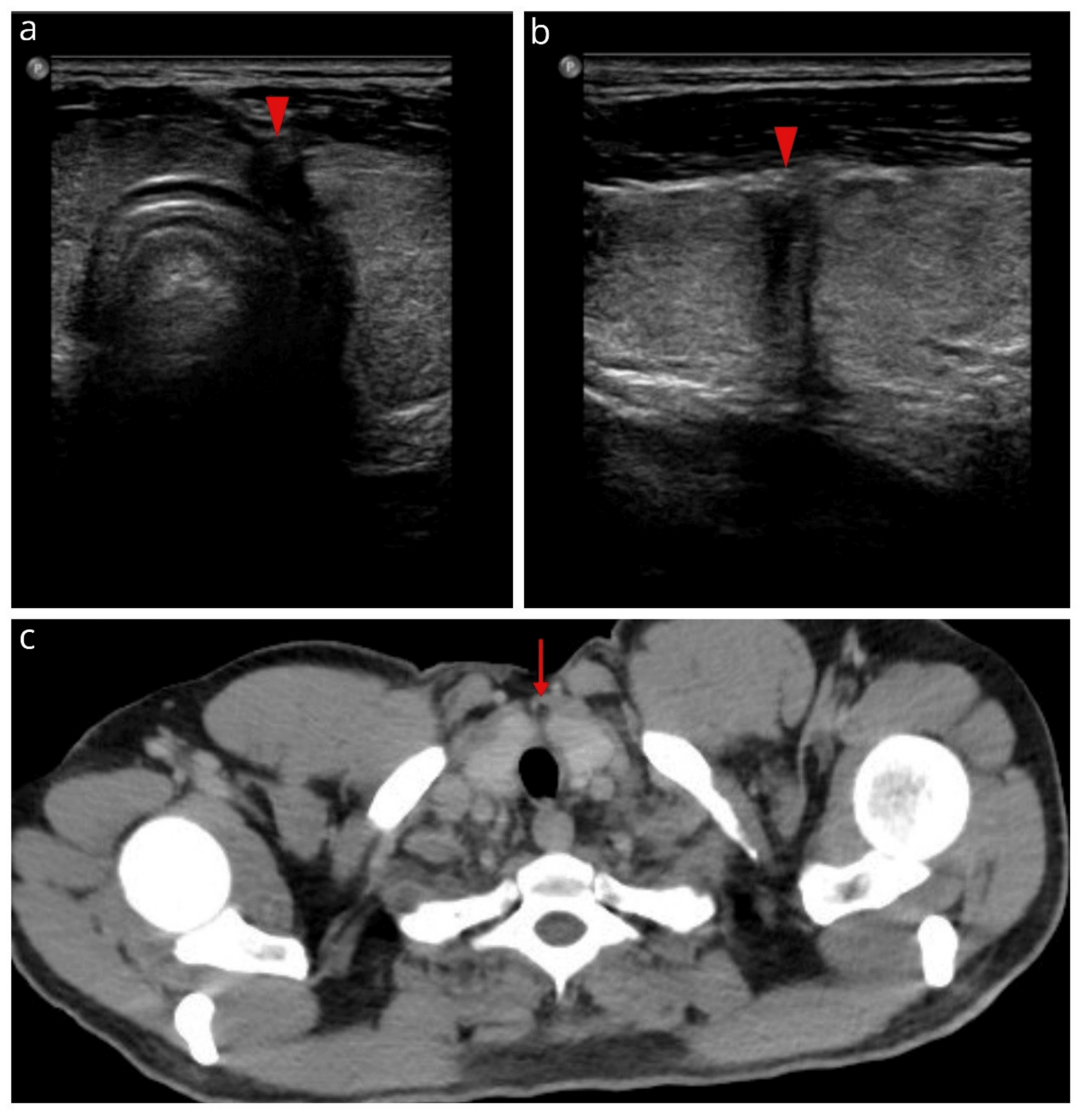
Two-month 2D B-mode ultrasound with transverse (a) and sagittal (b) views of the left thyroid lobe and an 18-month chest contrast-enhanced computed tomography (CECT) examination (c) Fibrous tissue is located at the level of the parenchymal rupture (arrowheads). CECT shows the parenchymal scar (arrow).
